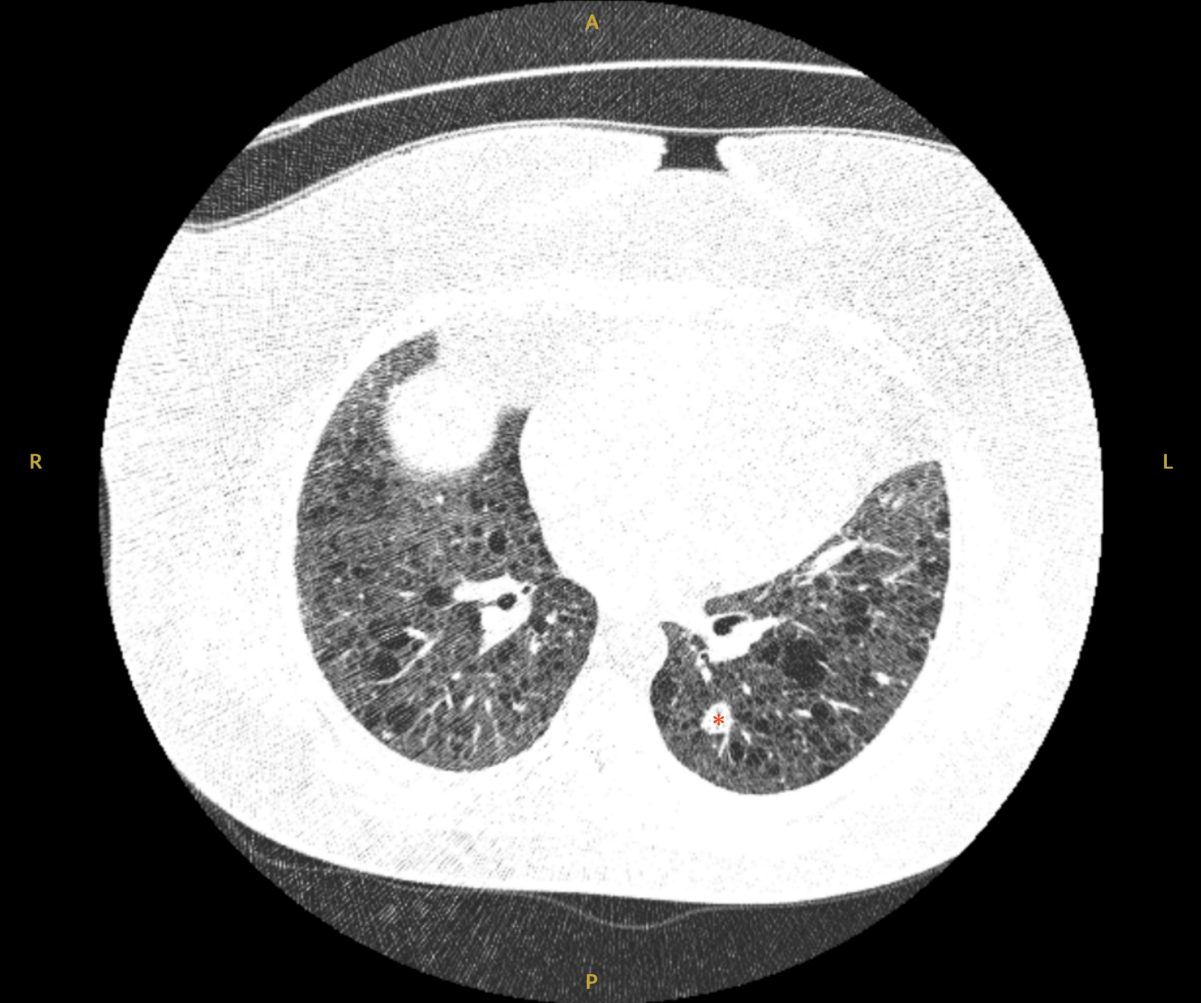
Cross-sectional axial high-resolution computed tomography image showing evidence of diffuse thin-wall cysts as well as a 22-mm multifocal micronodular pneumocyte hyperplastic lesion in the left lower lobe (*). A: anterior; P: posterior; R: right; L: left.
